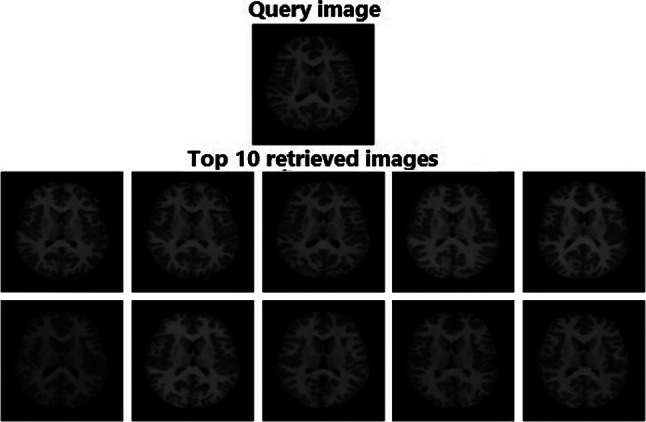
Retrieved images for an OASIS-MRI database query using RbQE with AlexNet
